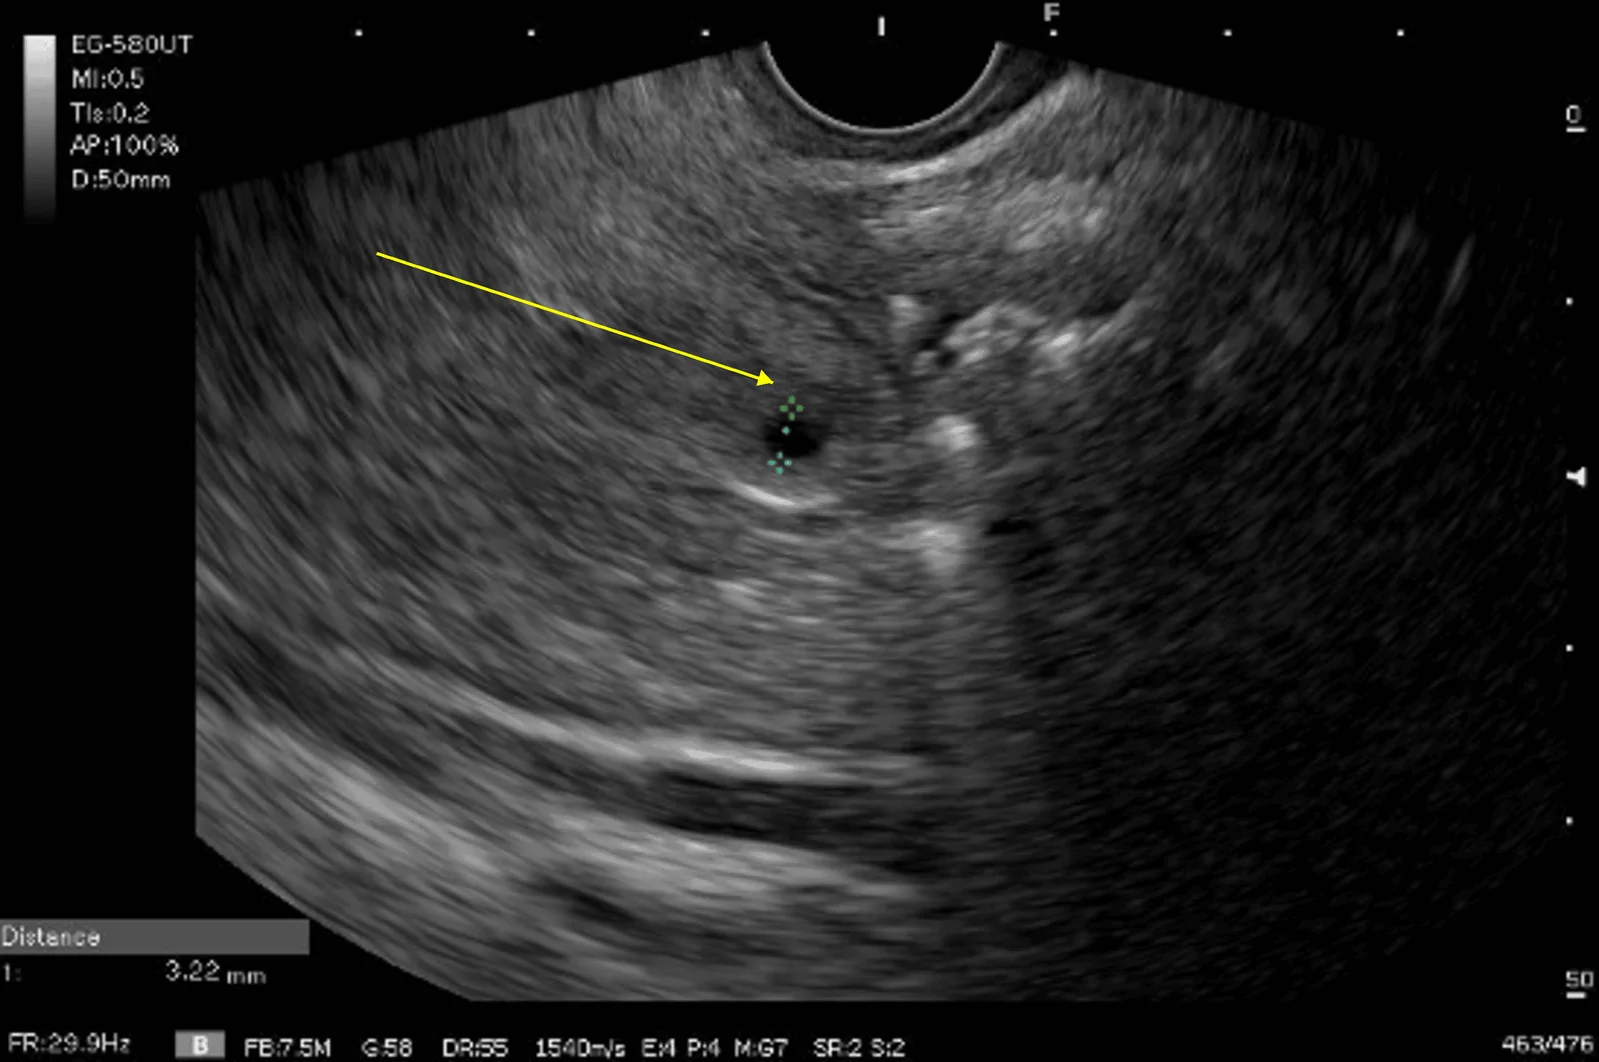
Endoscopic ultrasonography image showing a 3-mm intra-ampullary cystic lesion (arrow), corresponding to the wirsungocele.
